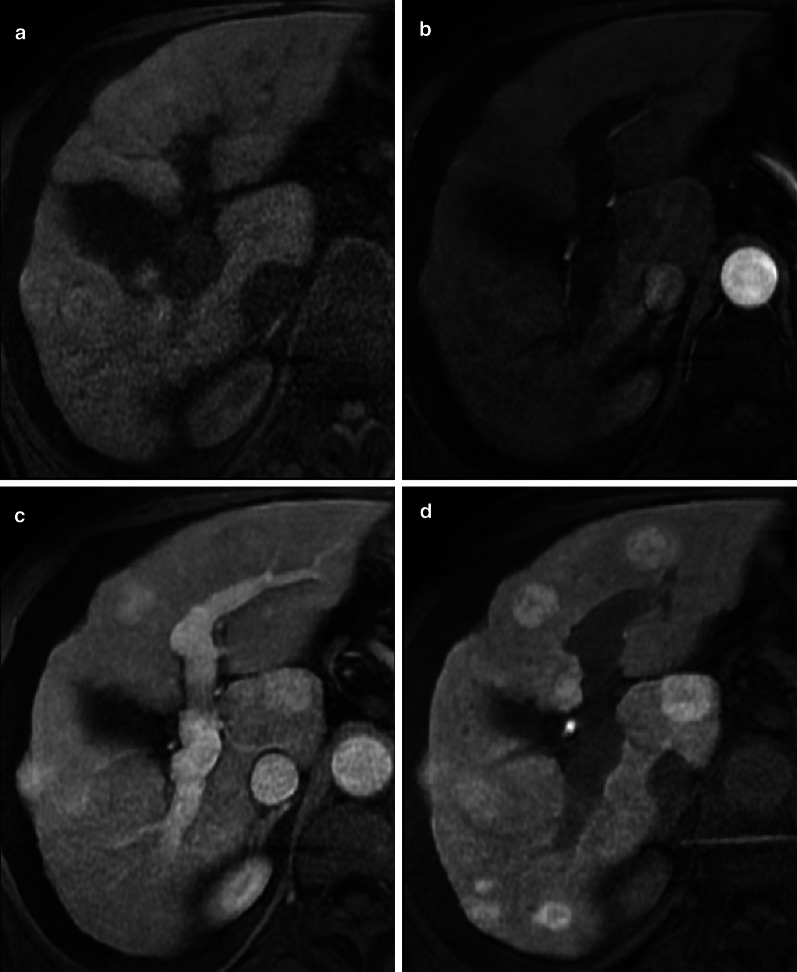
A 71-year-old man with HCV-related cirrhosis and multiacinar cirrhotic nodules. Gadoxetate disodium-enhanced MR shows multiple multiacinar cirrhotic nodules that are (a) isointense to surrounding liver parenchyma in the precontrast T1-weighted sequence and (b) in the hepatic arterial phase, (c) show enhancement in the portal venous phase due to early uptake of hepatobiliary contrast and (d) are hyperintense in the hepatobiliary phase
